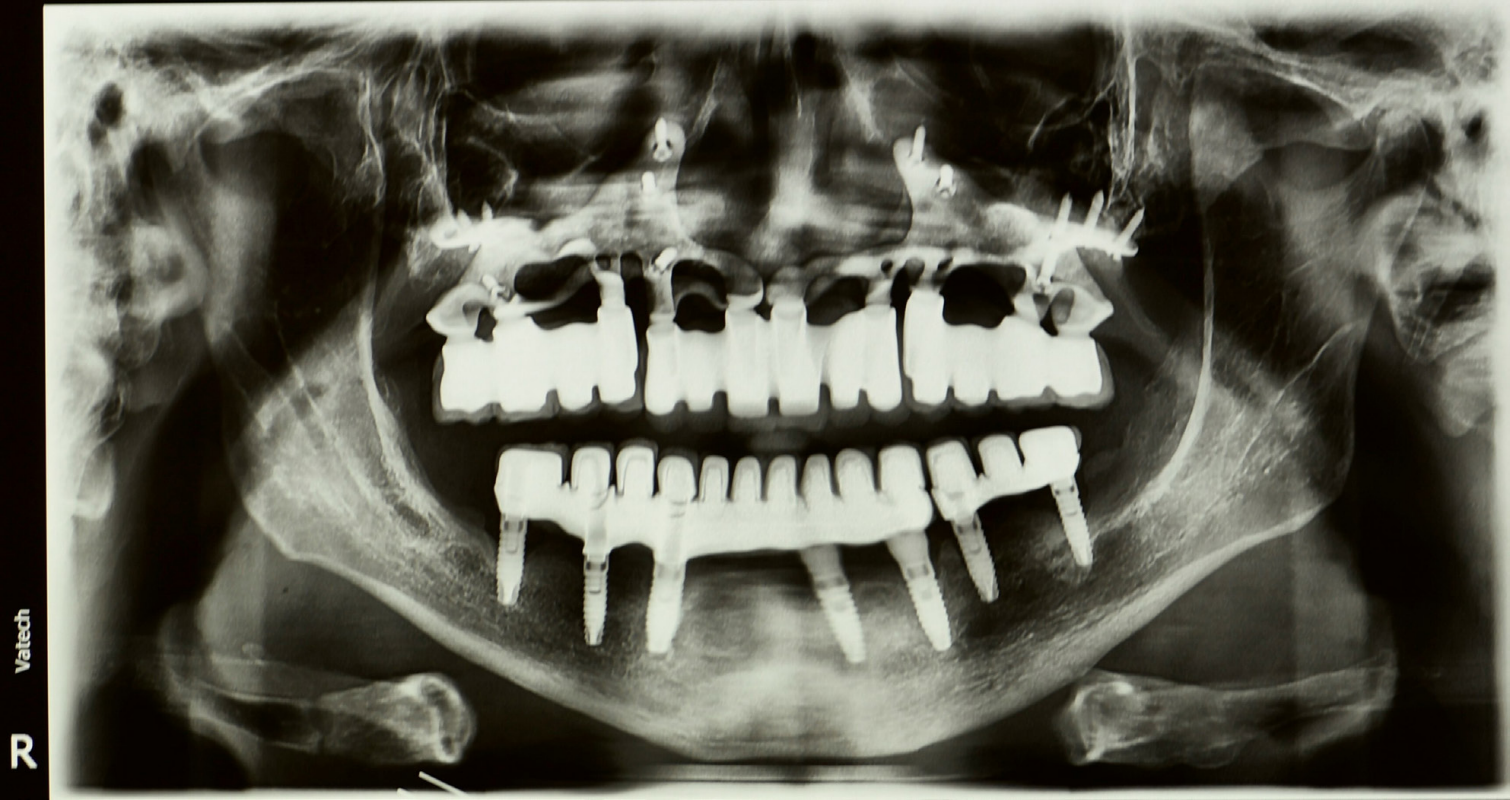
Panoramic radiography showing implant status after 3 years.
